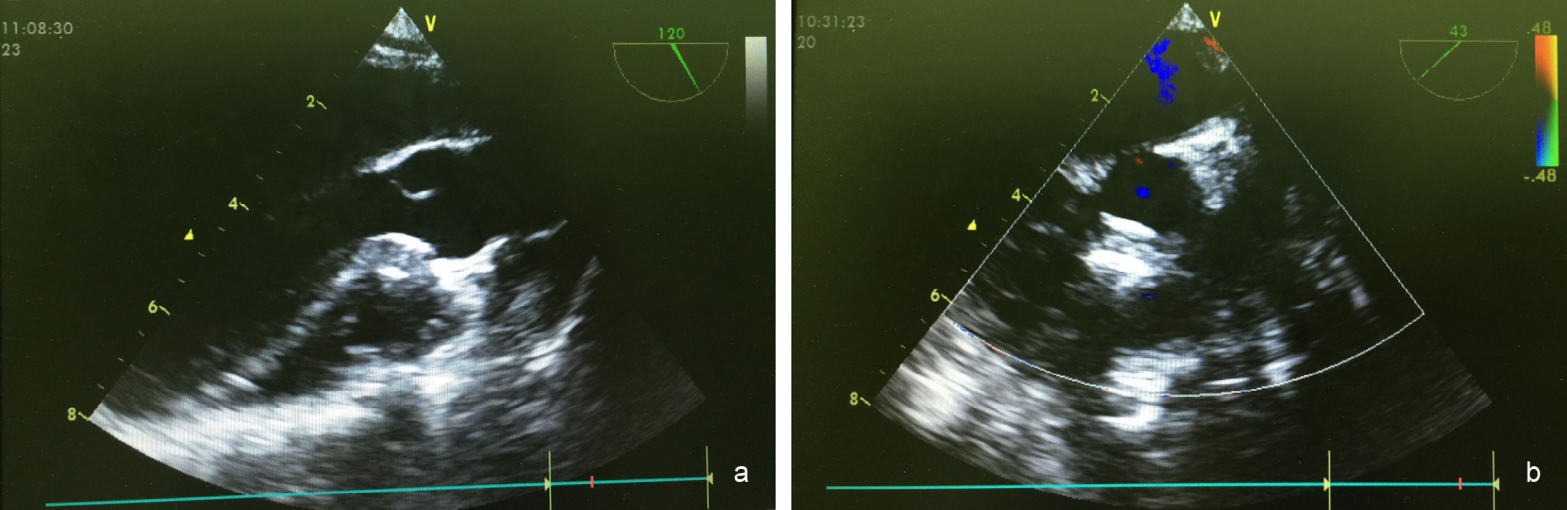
Transesophageal echocardiography after the procedure. A: long axis 2D view of the device. B: short axis of the device with color Doppler.
